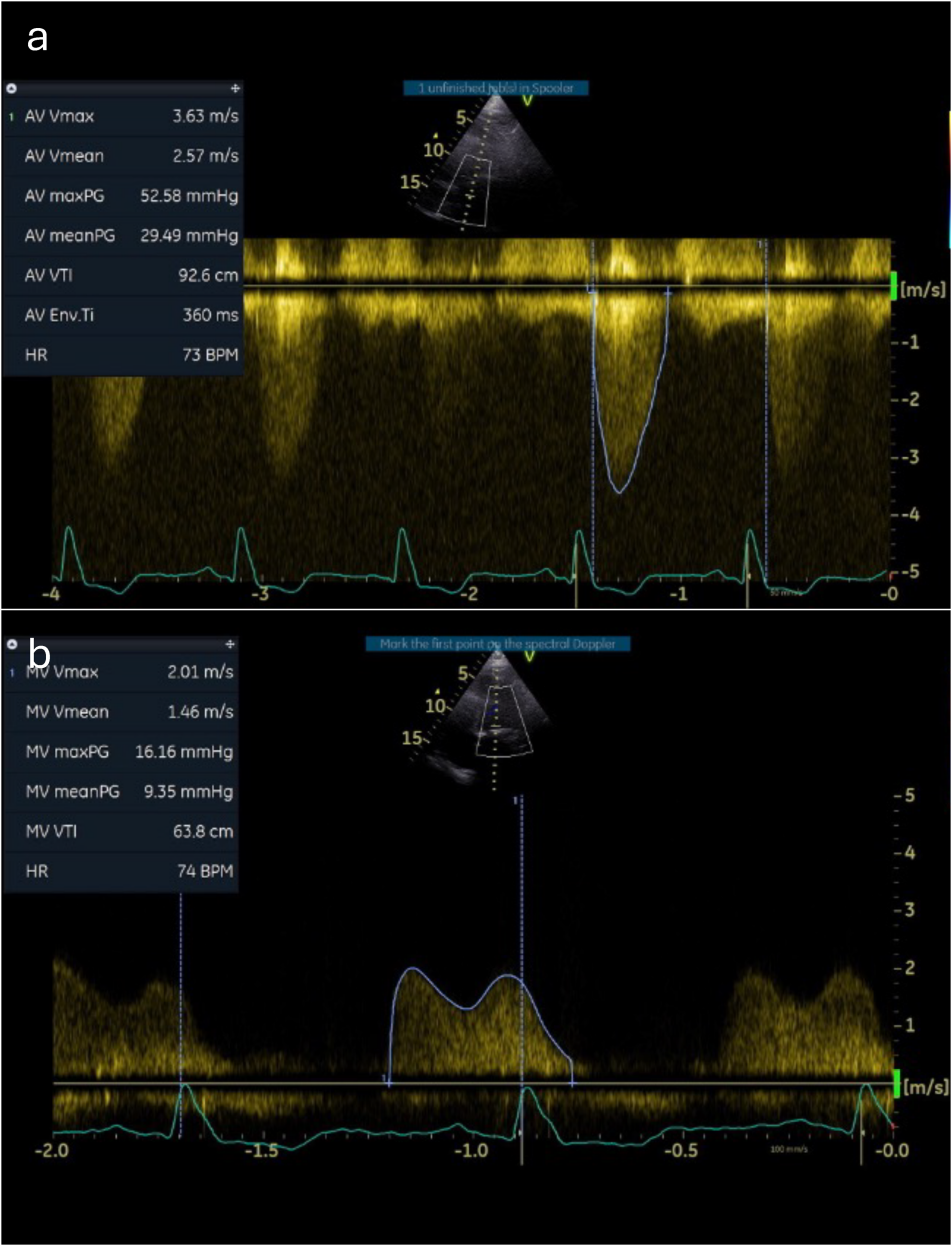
Baseline Doppler tracings across the aortic and mitral valves. Continuous-wave Doppler measurements through the aortic (a) and mitral (b) valves demonstrate severe stenosis.
